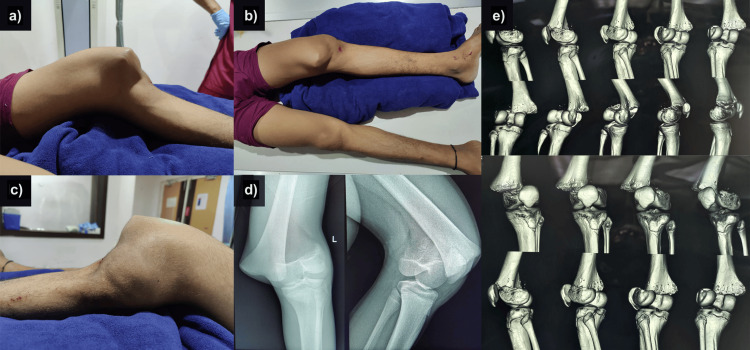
Clinical findings at the time of presentation (a-c); X-ray shows a Salter-Harris type II physeal slip (d); computed tomography scan showing the distal femoral physeal slip (e).
